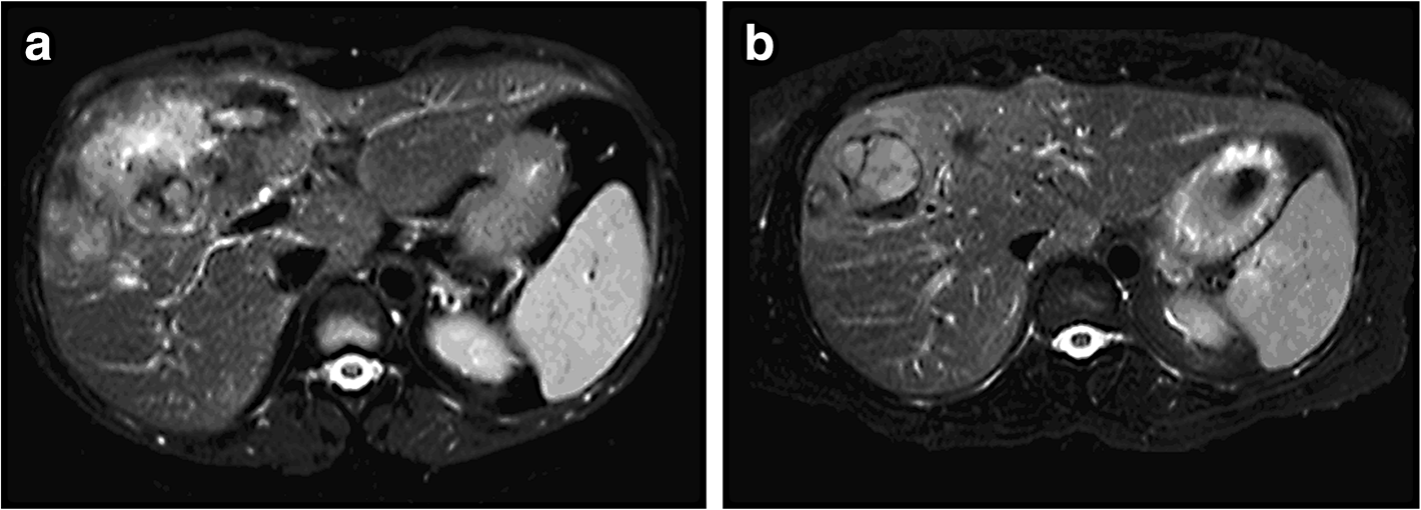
Liver MRI scans. (a) At first diagnosis. (b) After 8 months of sirolimus, showing a very good radiological response.
